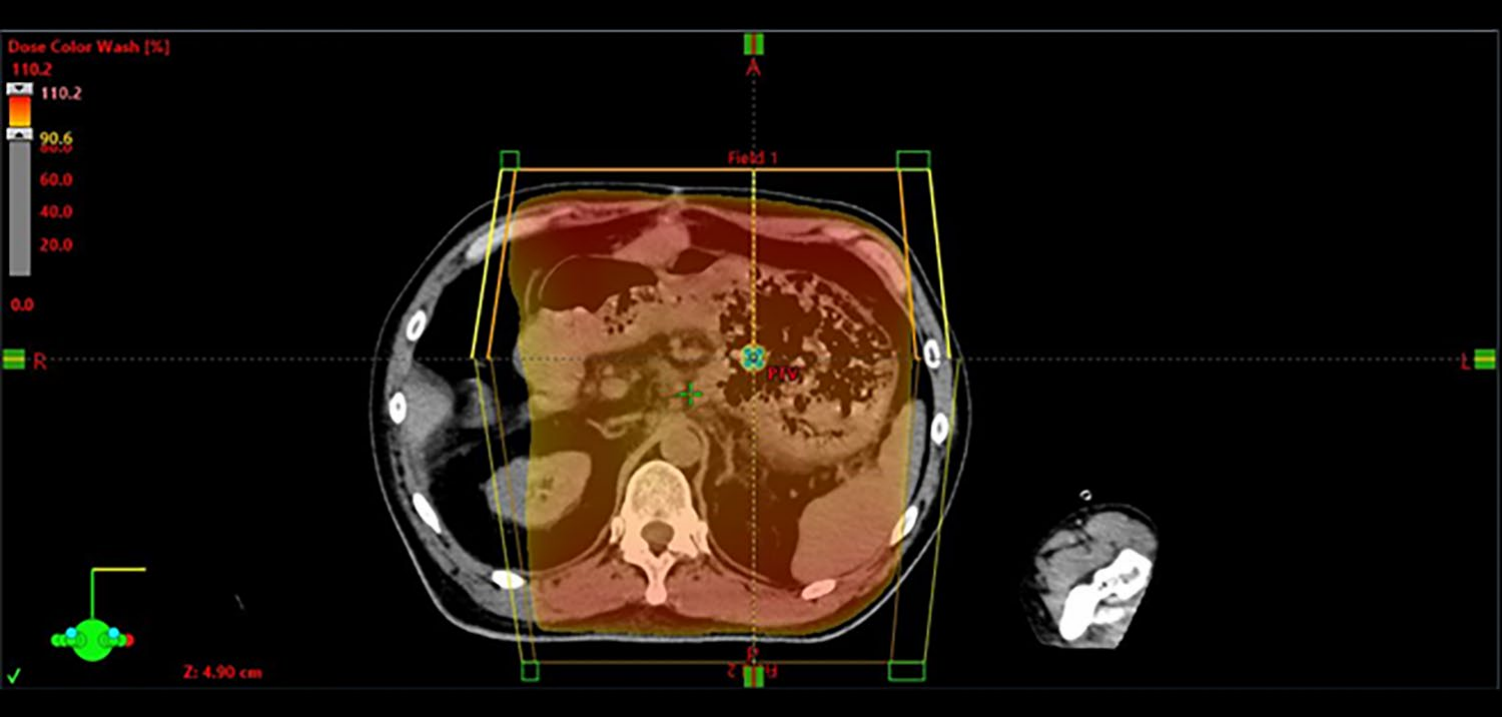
Key images of the hemostatic radiotherapy planning. Two opposed radiation fields from the anteroposterior direction were used, delivering 10 megavoltage X-rays of 8 Gy in a single fraction to the whole stomach
